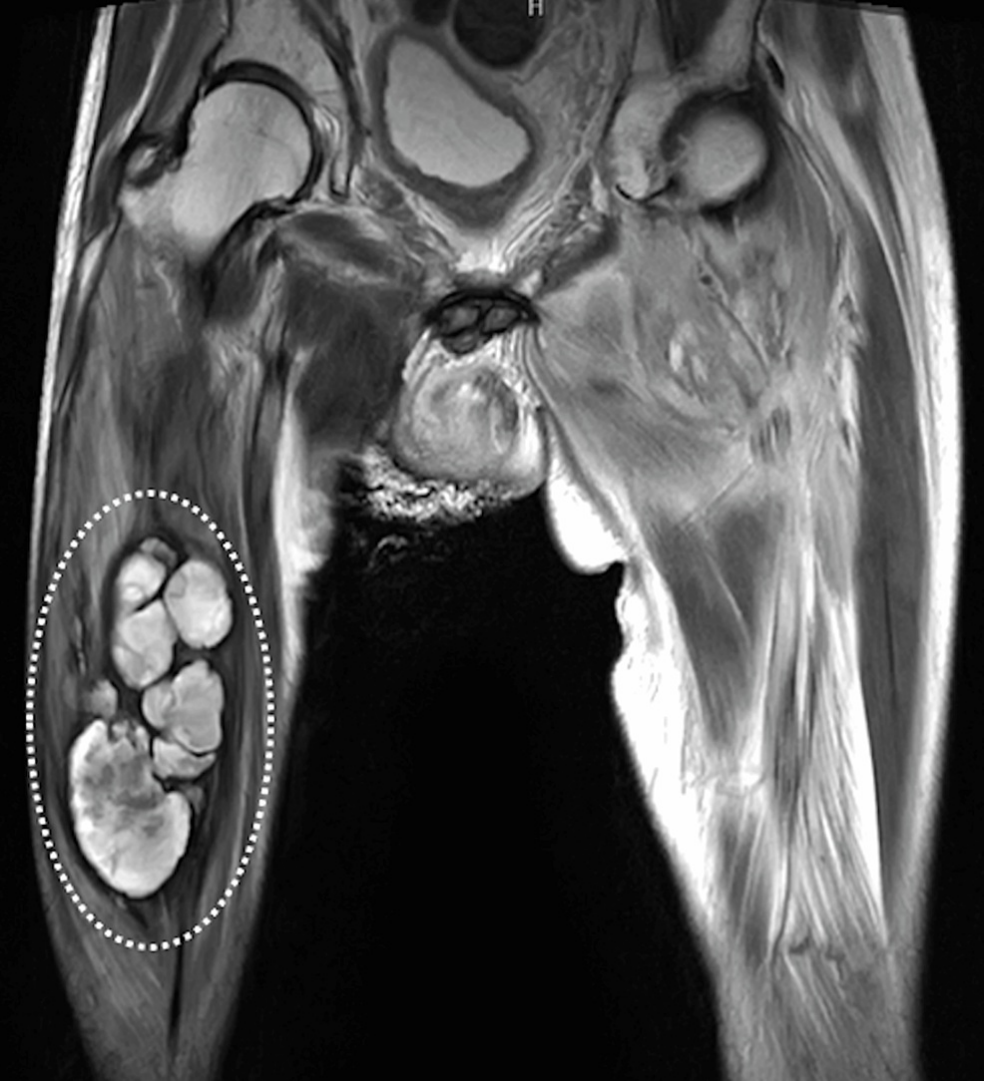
Intramuscular mass in the right thigh The dotted white oval shows an intramuscular mass in the right thigh on a T2-weighted magnetic resonance image.
